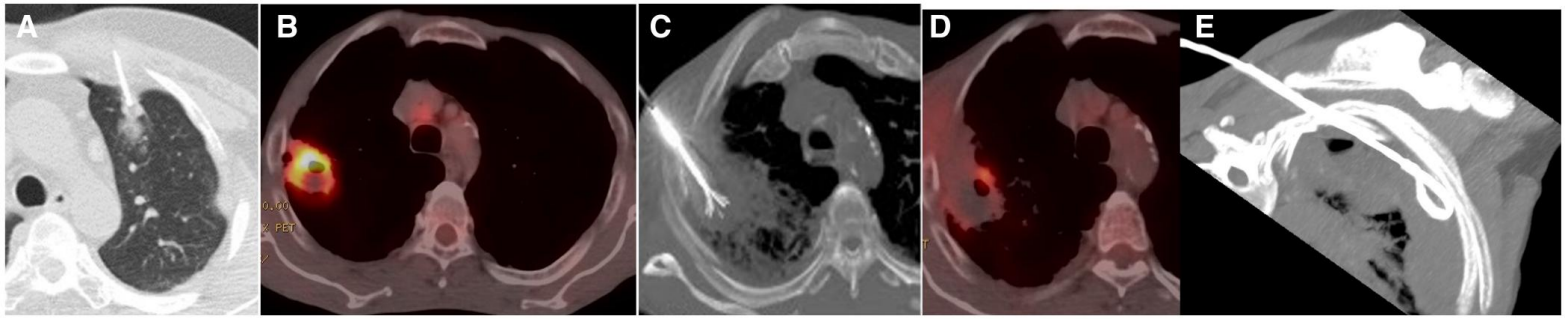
Role of interventional radiology in lung cancer management. (A) CT guided biopsy from left upper lobe lung adenocarcinoma done with oblique approach lateral to the left internal mammary artery with biopsy needle tip within the lesion. (B) Pre radiofrequency ablation (RFA) FDG PET CT shows metabolic activity within the lung cancer. (C) Position of RFA electrode within the lung lesion shown in B. (D) Post RFA FDG PET CT showing residual activity only at the periphery of the lesion in B. (E) Right pleural effusion after surgery for lung cancer with CT guided pigtail in situ.
